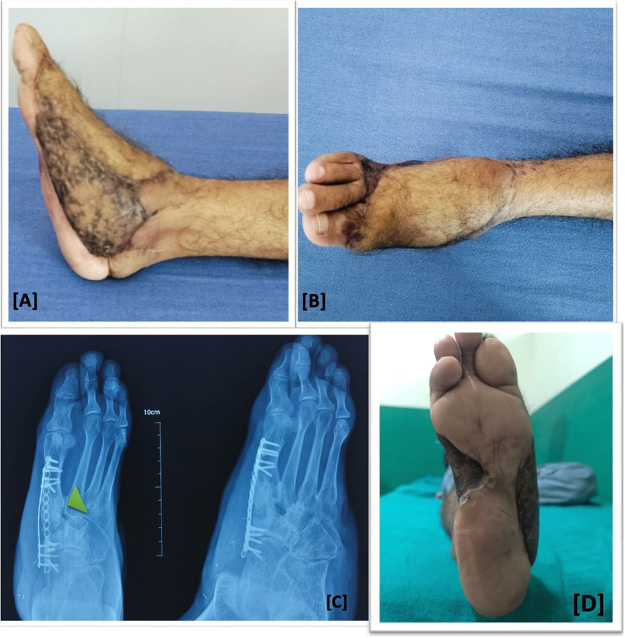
At 12-month follow-up: satisfactory aesthetic and functional recovery (A and B); plain radiograph showing complete union(arrowhead) with intact hardware (C); 17 months follow up of showing adequate normal skin at sole of foot with healed graft and flap (D).
